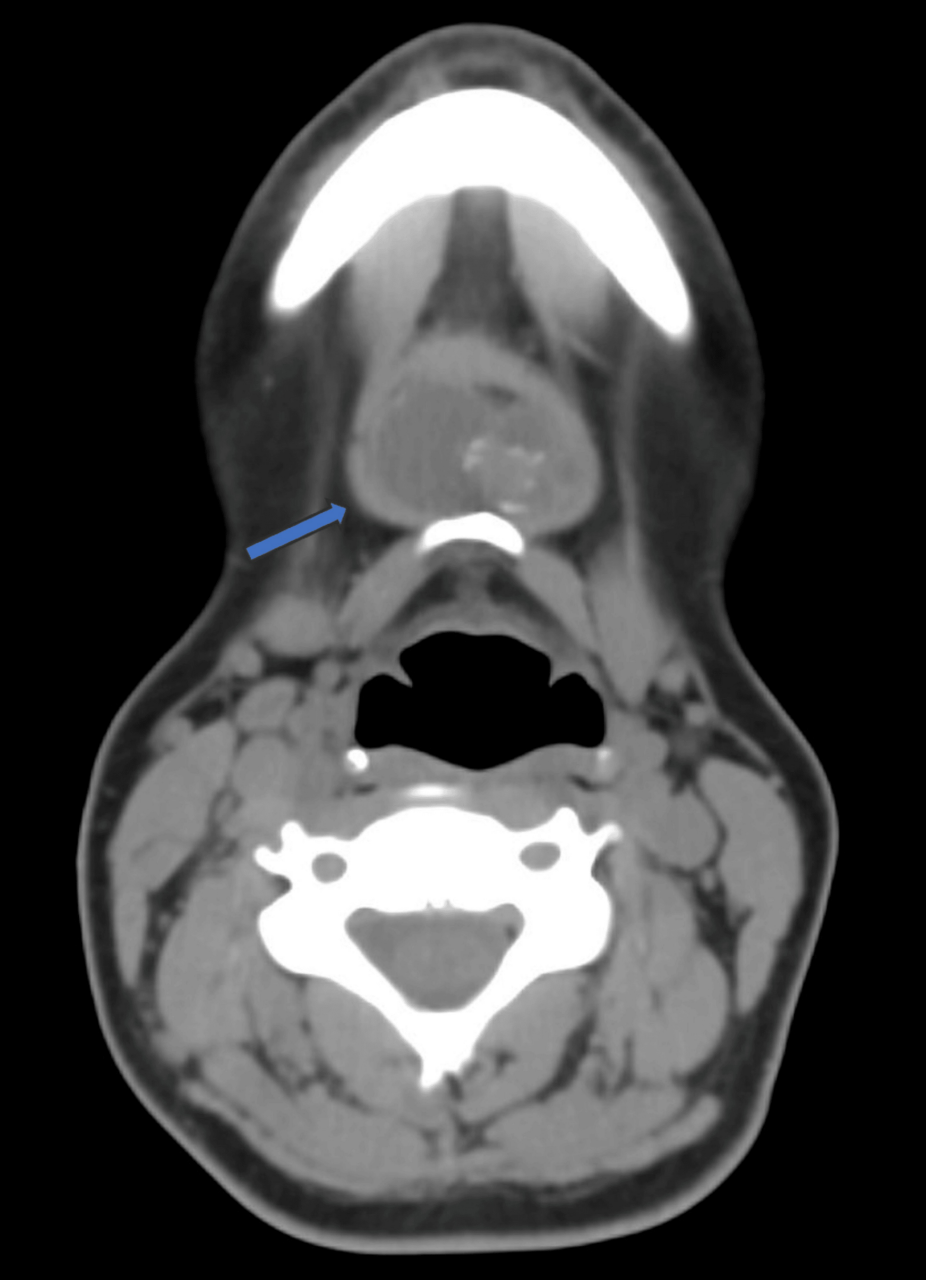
CT scan showing a cystic mass in the hyoid bone containing a solid component with calcifications CT: computed tomography
